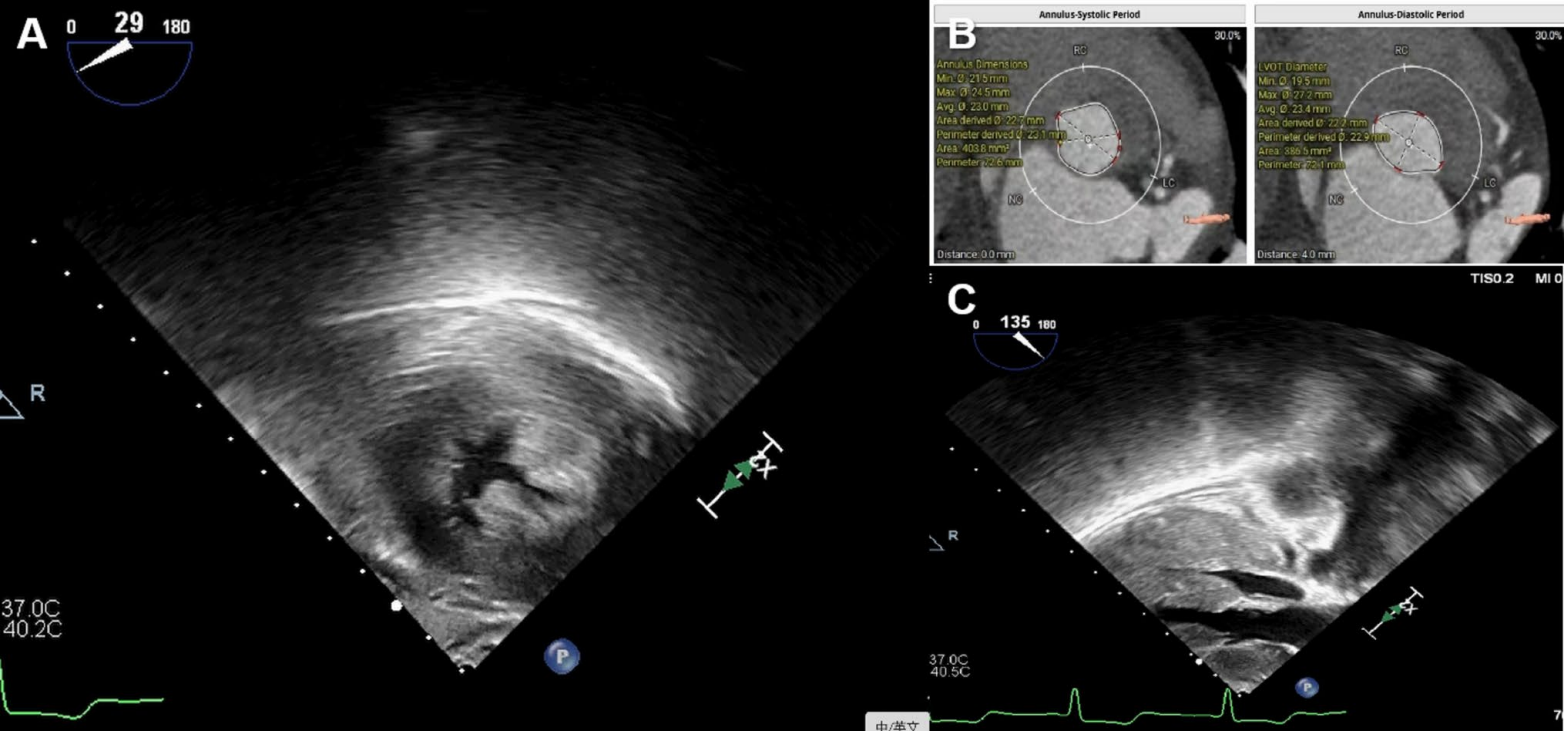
Transesophageal echocardiographic (TEE) and computed tomography (CT) images demonstrating aortic valve stenosis and hypertrophic obstructive cardiomyopathy (HOCM). A Shows restricted aortic valve opening with thickened cusps and dynamic left ventricular outflow tract obstruction due to asymmetric septal hypertrophy on TEE. B Presents CT-based measurements of the aortic annulus during diastole and systole, including annular diameters and areas, aiding in procedural planning. C Demonstrates the left ventricular outflow tract is narrowed due to asymmetric septal hypertrophy, consistent with dynamic obstruction
